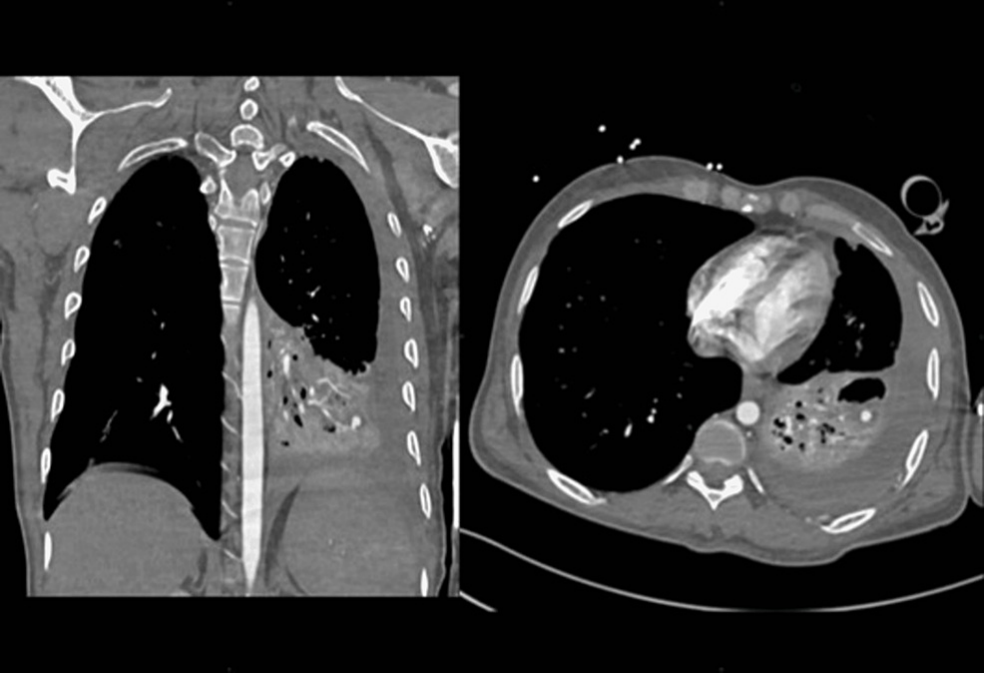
The CT pulmonary angiogram showing left lower lobe near complete collapse-consolidation with at least two cavitary lesions. Small pseudoaneurysm is noted measuring 5 x 6.6 x 5 mm arising from a subsegmental branch of the left lateral basal segmental artery of the left lower lobe adjacent to the cavitary lesion (Rasmussen aneurysm) in the background of pulmonary tuberculosis.
